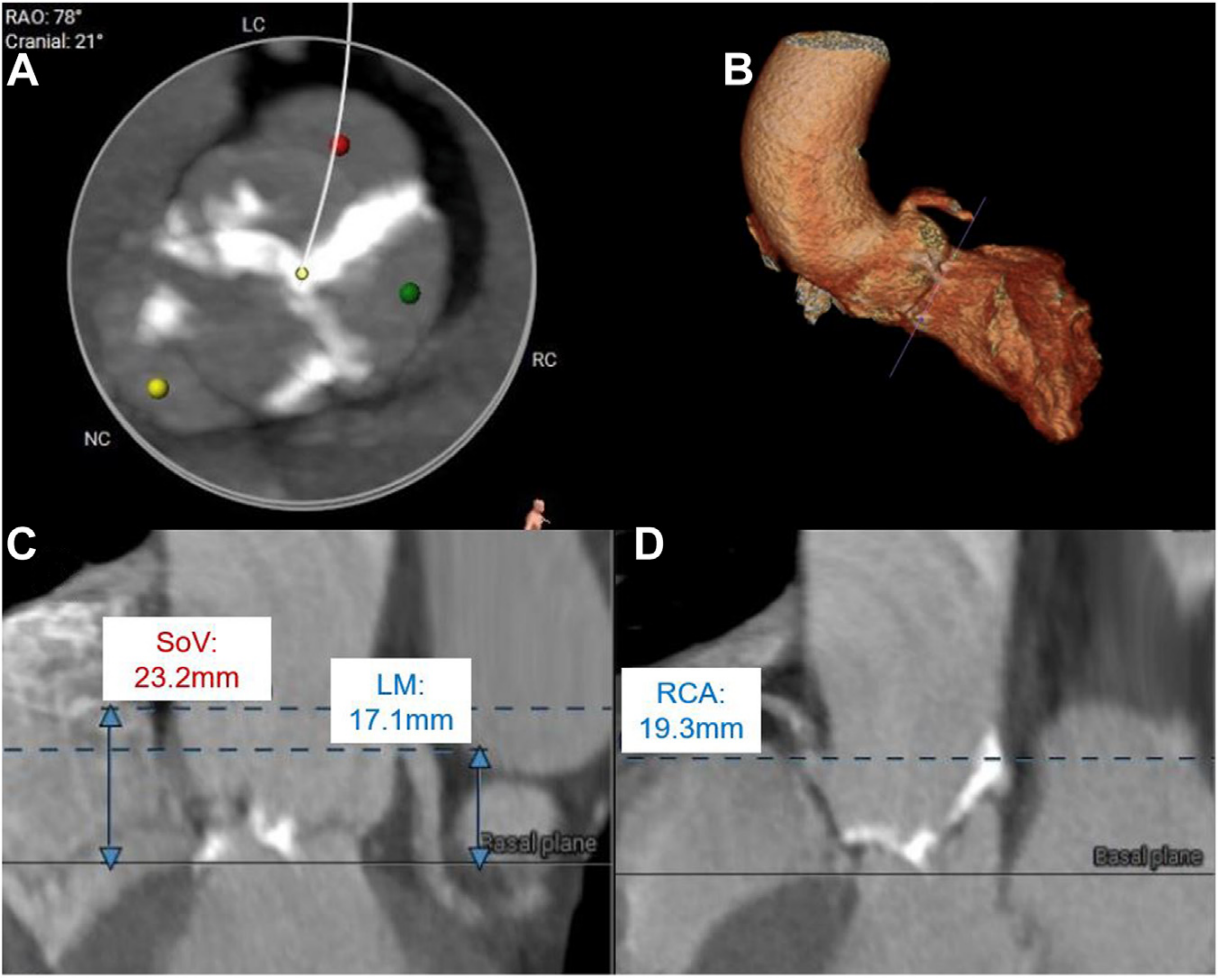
Cardiac CT with contrast. (A) Cardiac CT reveals a calcified fusion of the left and right coronary cusps. The aortic valve annulus is measured as 20 × 27 mm with an area of 419 mm^2^ and a circumference of 47 mm with an aortic valve calcium score of 2798. (B) Three-dimensional reconstruction of the sinuses of Valsalva. The origin of the left coronary artery from the sinus of Valsalva is seen. (C) Left coronary artery to annulus height of 17 mm. Sinus of Valsalva height is measured as 23 mm with a width of 25 × 27 × 33 mm. (D) Right coronary artery to annulus height of 19 mm. CT, computed tomography.
